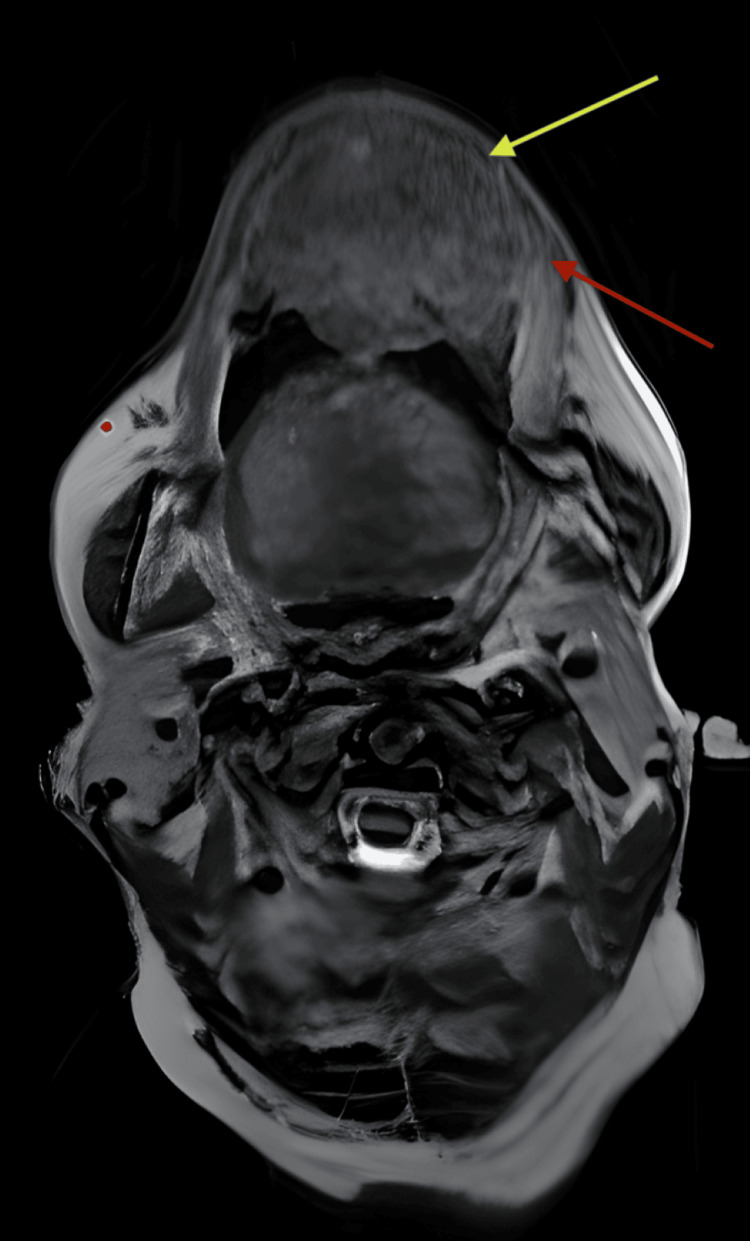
Contrast-enhanced MRI of an infiltrative mandibular alveolar ridge tumor Axial contrast-enhanced MRI shows a large, expansile lesion centered in the left mandibular alveolar ridge, extending across the midline into the right alveolar ridge. The mass demonstrates homogeneous enhancement, diffusion restriction on diffusion-weighted imaging (DWI) with corresponding low apparent diffusion coefficient (ADC) values, and measures approximately 62 × 44 mm (yellow arrow). There is associated cortical bone destruction with loss of mandibular continuity (red arrow). Adjacent soft tissue involvement is noted.
